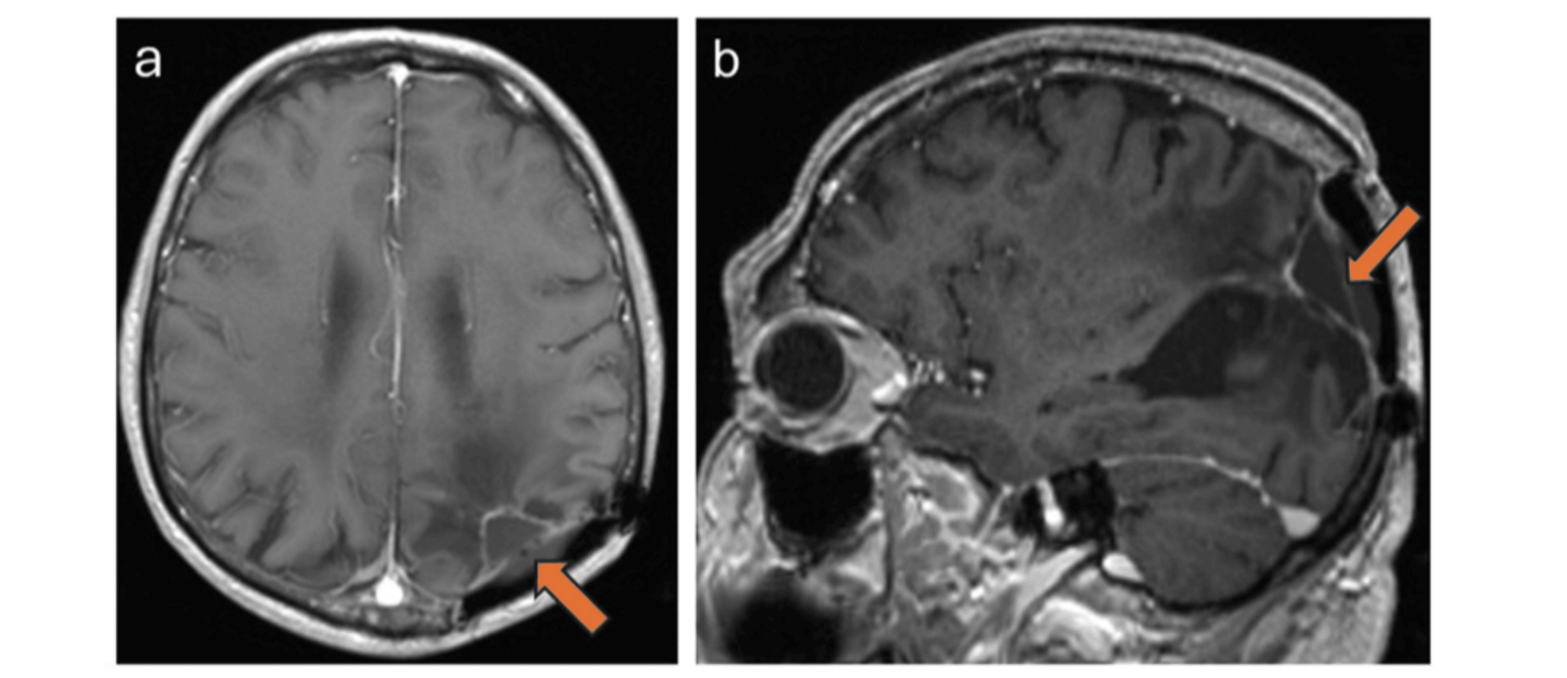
Radiographic imaging five years after surgery and radiation therapy. (a) Axial T1-weighted MR image with contrast and (b) sagittal T1-weighted MPRAGE image showing postoperative and post-radiation changes in the left parietal lobe. Note the contrast enhancement at the margins of the resection cavity (arrow).
